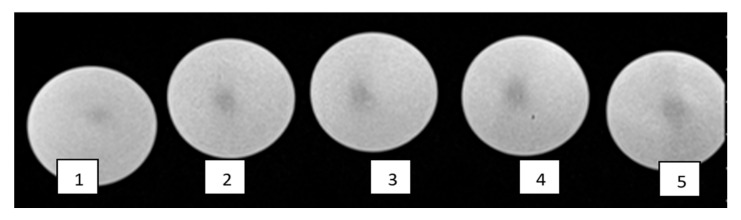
Scanned MRI images of dose gels irradiated with: 1—10 Gy, 2—13 Gy, 3—18.6 Gy, 4—26 Gy and 5—43.3 Gy.
